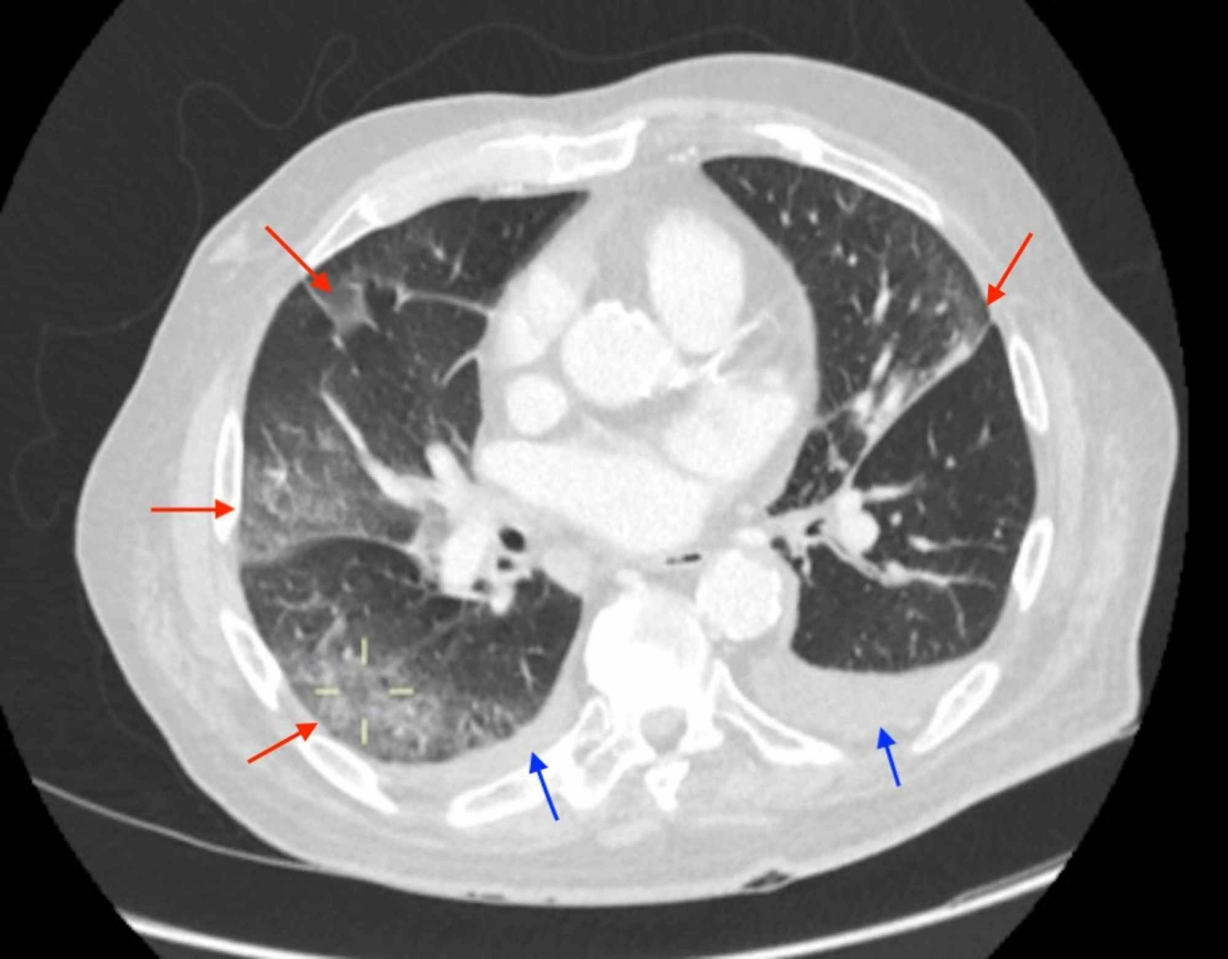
CT Chest - Axial View Peripheral ground-glass opacification within the right upper and lower lobes and the left upper lobe (red arrows). Minimal changes were noted within the lingula. There were small bilateral pleural effusions (blue arrows). These appearances were considered equivocal for COVID-19 infection.
